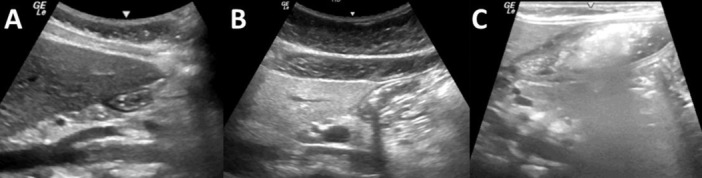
Figure 1. Representative images of point‐of‐care gastric ultrasound findings (A) empty, Patient 13 (B) clear liquid, Patient 9 (C) solid‐appearing, Patient 3
